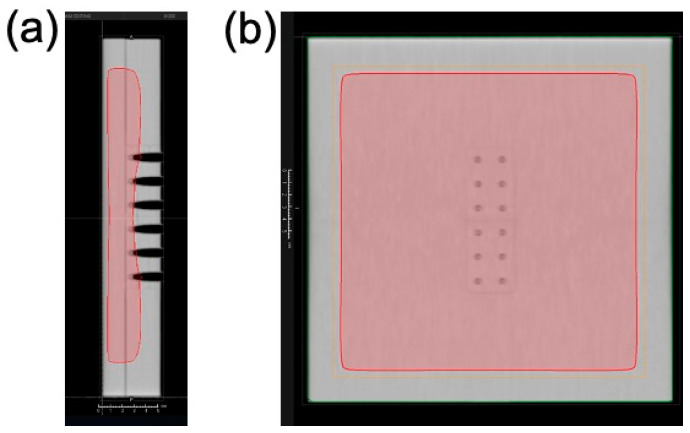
Dose distribution around the sample tube irradiated with 6 MV X-rays. Dose distribution (4 Gy) is presented in the color wash (red) from the side view (a) and the front view (b). Dose distribution was calculated using the treatment planning system.
